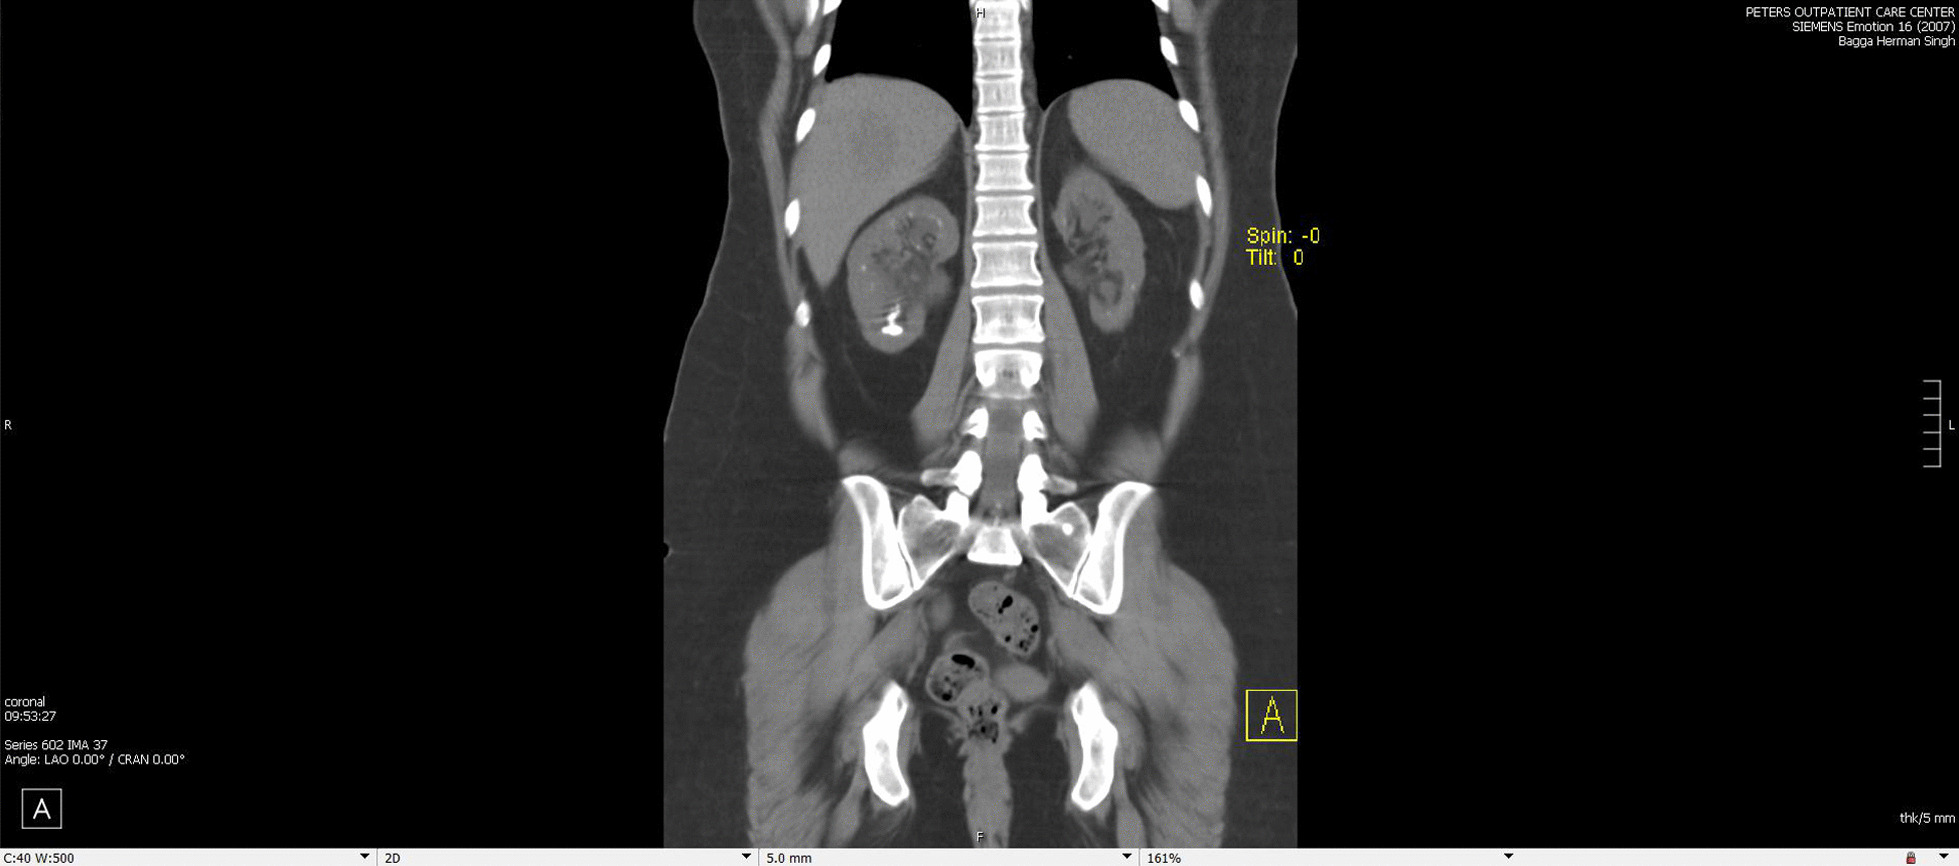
CT scan of patient in 2017 noting large right sided stones, nephrocalcinosis and left renal atrophy and dilated renal pelvis attributed to prior obstruction and surgical reimplantation of ureter done a few years earlier
